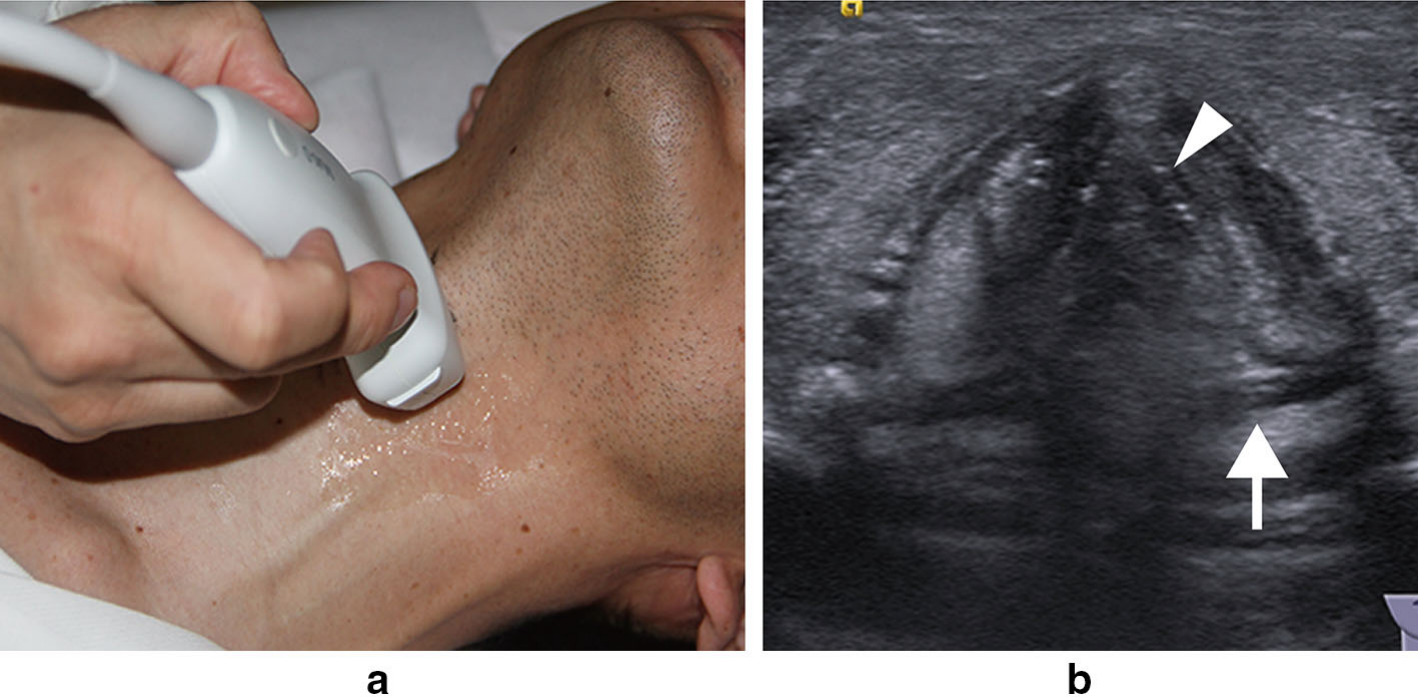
a Probe placed transversely over the middle portion of the thyroid cartilage. b Ultrasonographic view showing a normal visualized case. True vocal cord (*arrowhead*); arytenoid cartilage (*arrow*)
